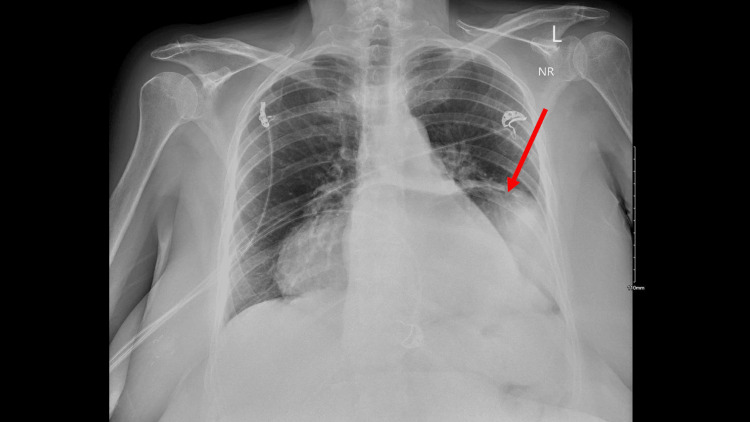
Bedside chest X-ray taken on hospital day 1 Findings: a large diaphragmatic/gastric hernia with distention of the stomach
